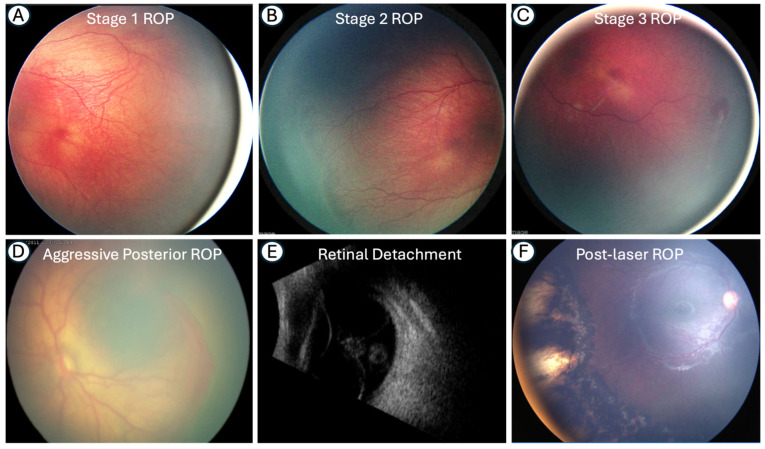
Clinical images demonstrating the progression of ROP through stages 1–3 (A–C), as well as A-ROP (D) and retinal detachment on ultrasound (E), along with a post-laser treatment image (F).
